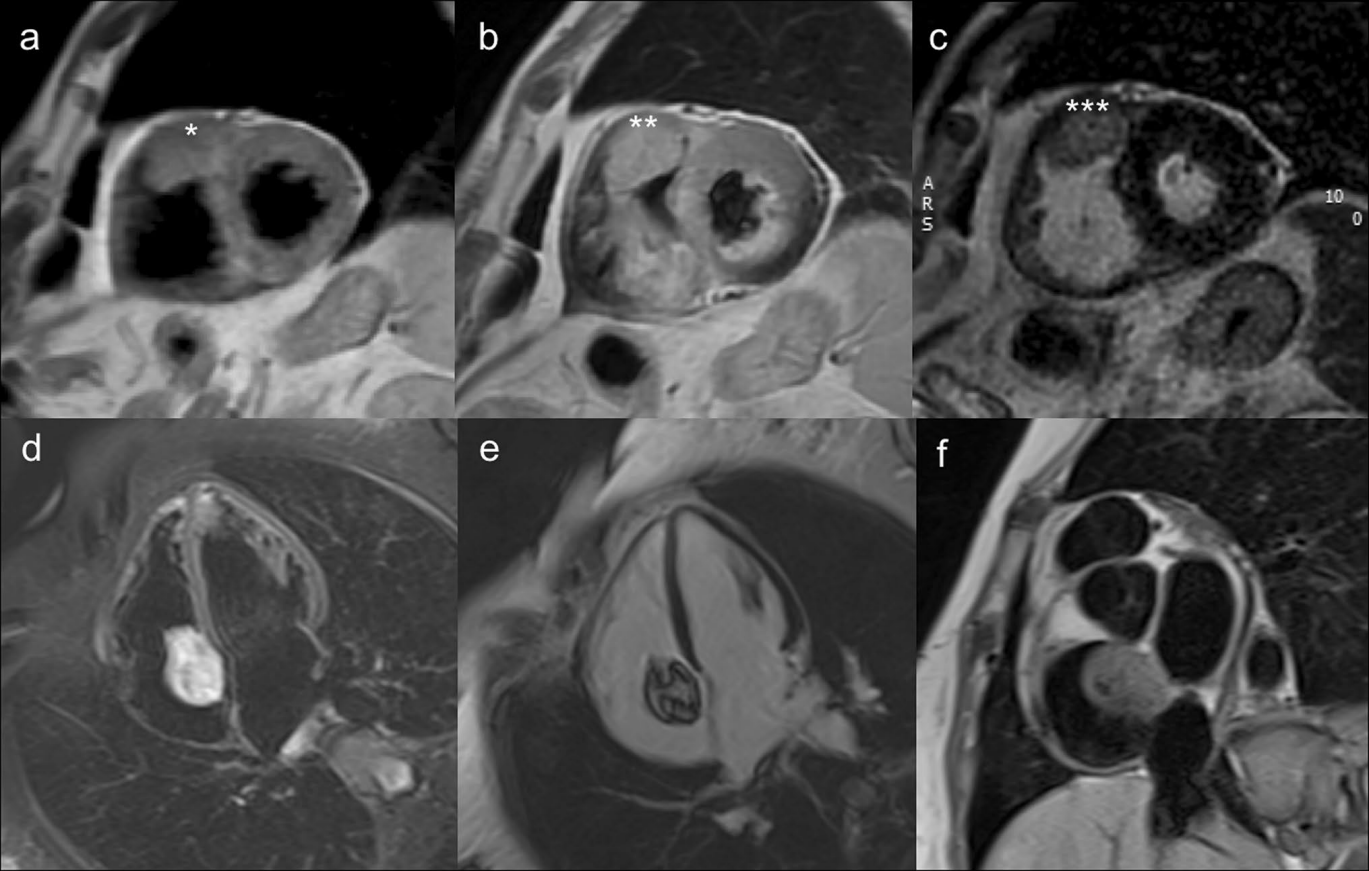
Examples of a typical malignant (angiosarcoma: a–c) and benign (myxoma: d–f) cardiac mass. a–c Angiosarcoma appears as a large, infiltrative lesion attached to the right atrial roof and extending in the pericardial space (*). There is inhomogeneous contrast enhancement in post-contrast T1-weighted and LGE images (b–c) as compared to pre-contrast T1-weighted short axis image  (*). Atrial myxoma on T1 weighted short axis image (d), shows spotty low signal intralesional components, consistent with presence of intratumoral calcifications (*). Lesion appears typically hyperintese in T2 weighted images (e), due to the myxoid tissue content of the mass. LGE sequences show inhomogeneous enhancement of the tumor  (f). LGE: Late Gadolinium Enhancement
